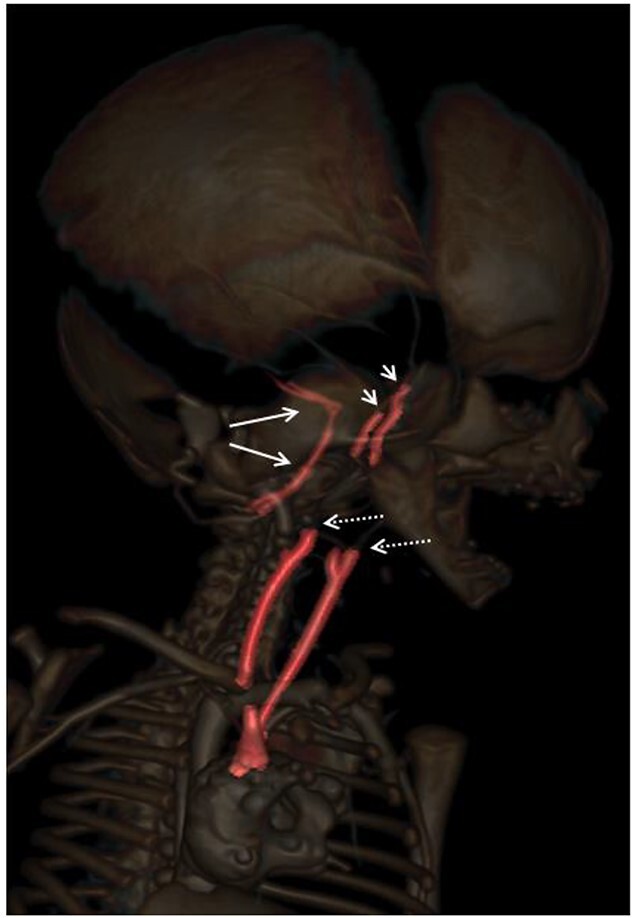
Whole body postmortem computed tomography angiography (PMCTA) (volume rendering, VR) lateral view of the head and antero-lateral view of the neck and superior part of the thorax, showing a full opacification of the vertebral arteries and basilar trunk with both posterior inferior cerebellar arteries (long white arrows), and the absence of opacification of the cervical terminal part of both internal carotid arteries (white spotted arrows). Intra-cranial carotid arteries, in their cavernous portions are partially visible/opacified (short white arrows).
